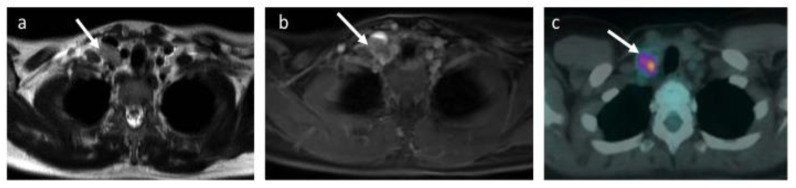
Anatomical boundary descriptive error. Images of a 31-year-old female patient with Ann Arbor stage II Hodgkin’s lymphoma. Patient had bulky mediastinal disease (not shown here) and right-sided supraclavicular nodal involvement. (a) T2-TSE, (b) contrast-enhanced water-only mDixon and (c) fused ^18^F-FDG PET-CT. The right supraclavicular node (arrows) is shown on a, b and c but was considered as supraclavicular on WB-MRI and deep cervical on ^18^F-FDG PET-CT. Following consensus read, the nodal station discrepancy was considered as anatomical boundary descriptive error.
